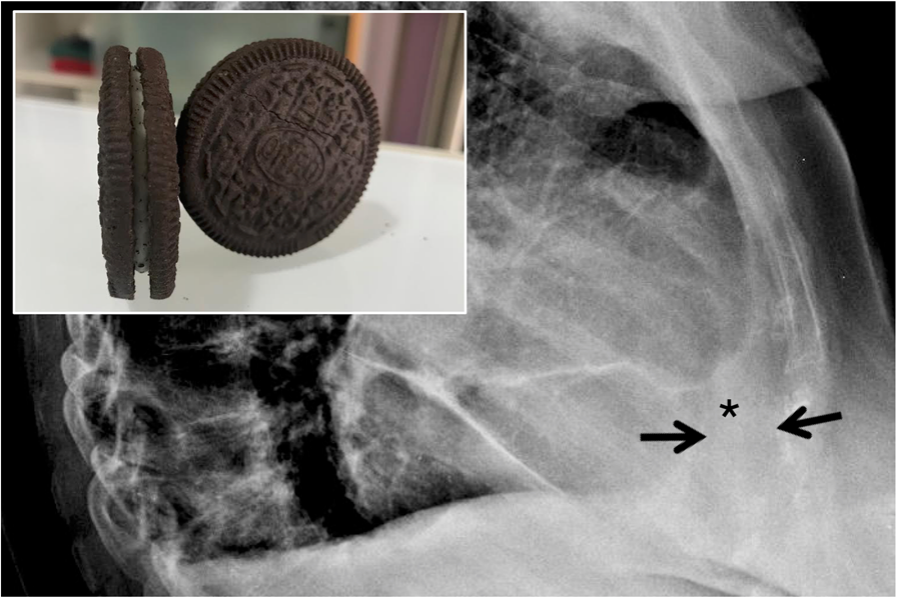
Oreo cookie sign. On lateral chest radiograph the Oreo cookie sign is depicted: it consists of an increased radiopacity of the pericardium (black asterisk)—caused by pericardial effusion—which appears to be anteriorly and posteriorly delimited by two radiolucent lines (black arrows), caused by pericardial and epicardial fat. This radiographic pattern reproduces the so called “Oreo cookie sign,” similar to the famous biscuit
